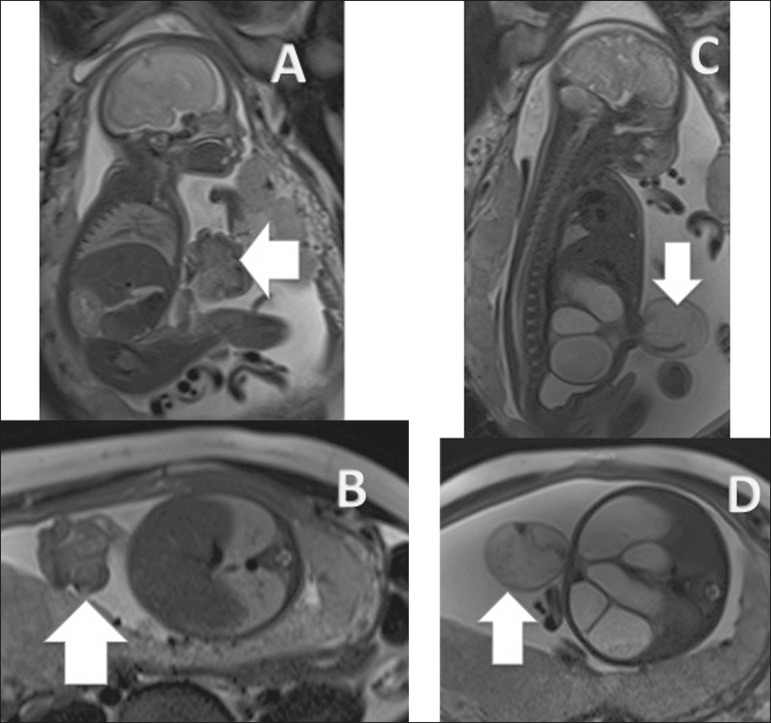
Gastroschisis. A,B: Sagittal T2-weighted sequence of a
